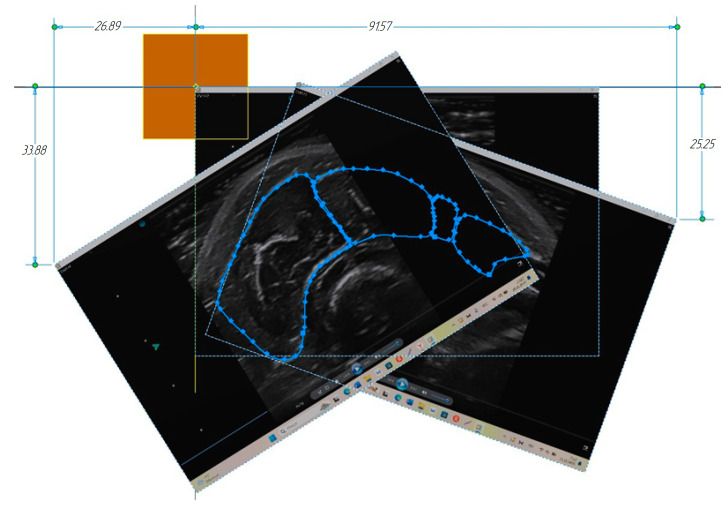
Contours of the forearm muscles under study when combined with the ultrasound images.
